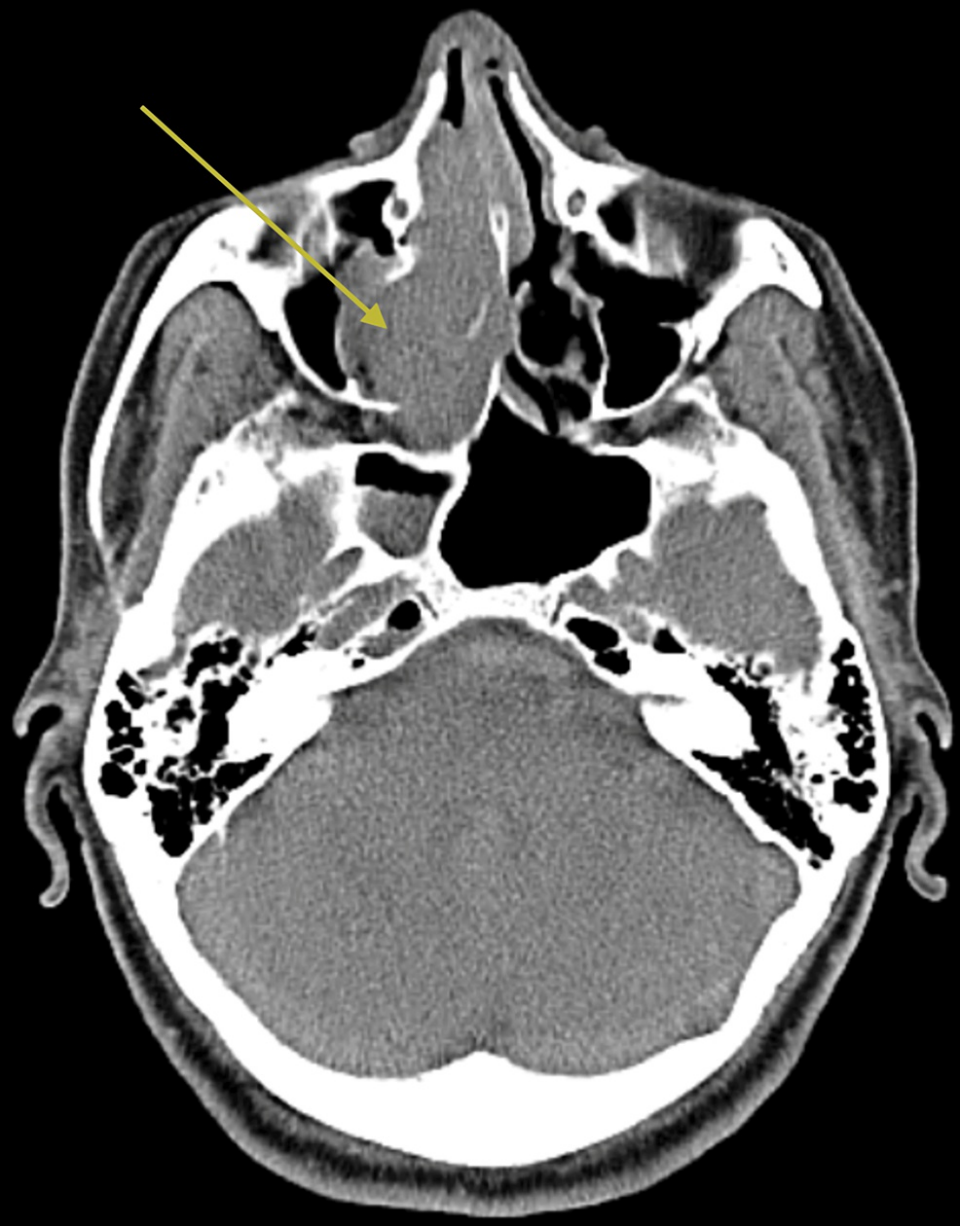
CT showing the soft-tissue density of the right side of the nasal cavity and also the widening of the osteomeatal complex (yellow arrow) CT: computed tomography
